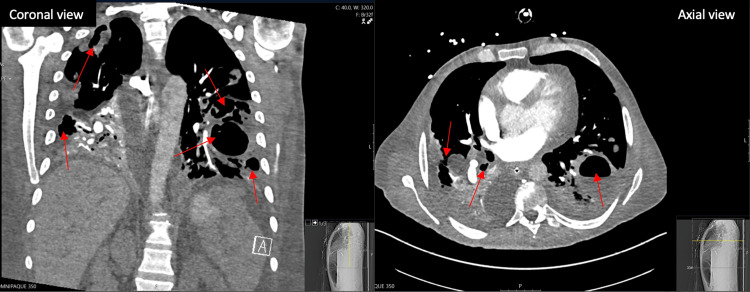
Chest CT angiography with contrast in the coronal and axial views 10 days after admission showing no signs of pulmonary embolism and worsening cavitary lesions Red arrows indicate cavitary lesions.
